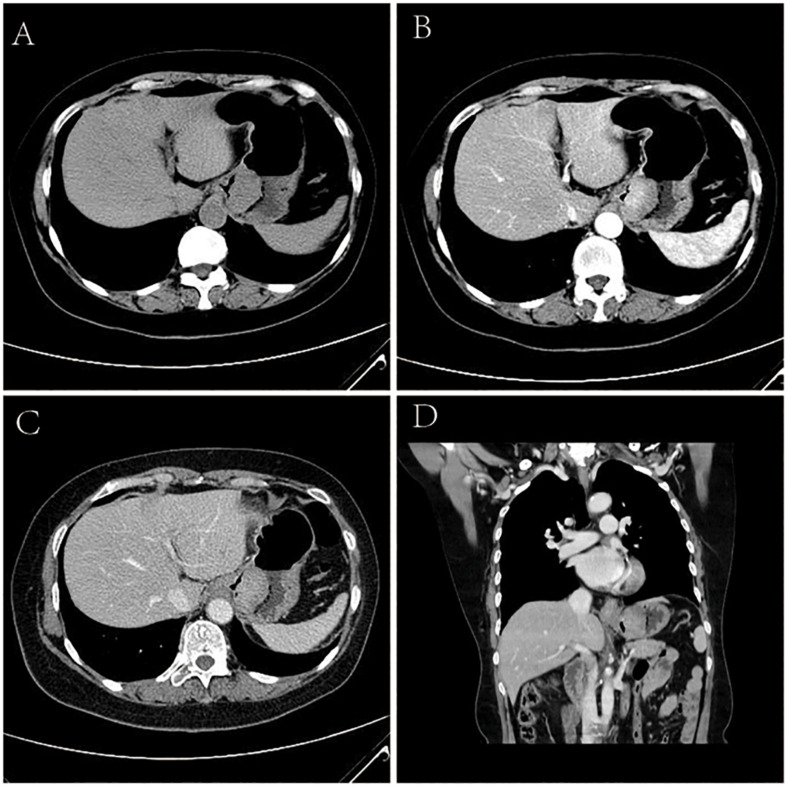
Sarcomatoid carcinoma of the stomach in 62-year-old women. (A) Unenhanced CT image of stomach reveals an intraluminal mass of homogeneous attenuation, with an irregular surface, at the gastric fundus and cardiac region. (B–D) Contrast-enhanced CT image shows obvious homogeneous enhancement of mass, with the peak value of the tumor on the portal phase. In perigastric lymph nodes, an enlarged and significantly enhancement lymph node can be seen. (B) Arterial phase of contrast enhancement image. (C) Portal phase of contrast enhancement image. (D) Portal phase of contrast enhancement coronal image.
